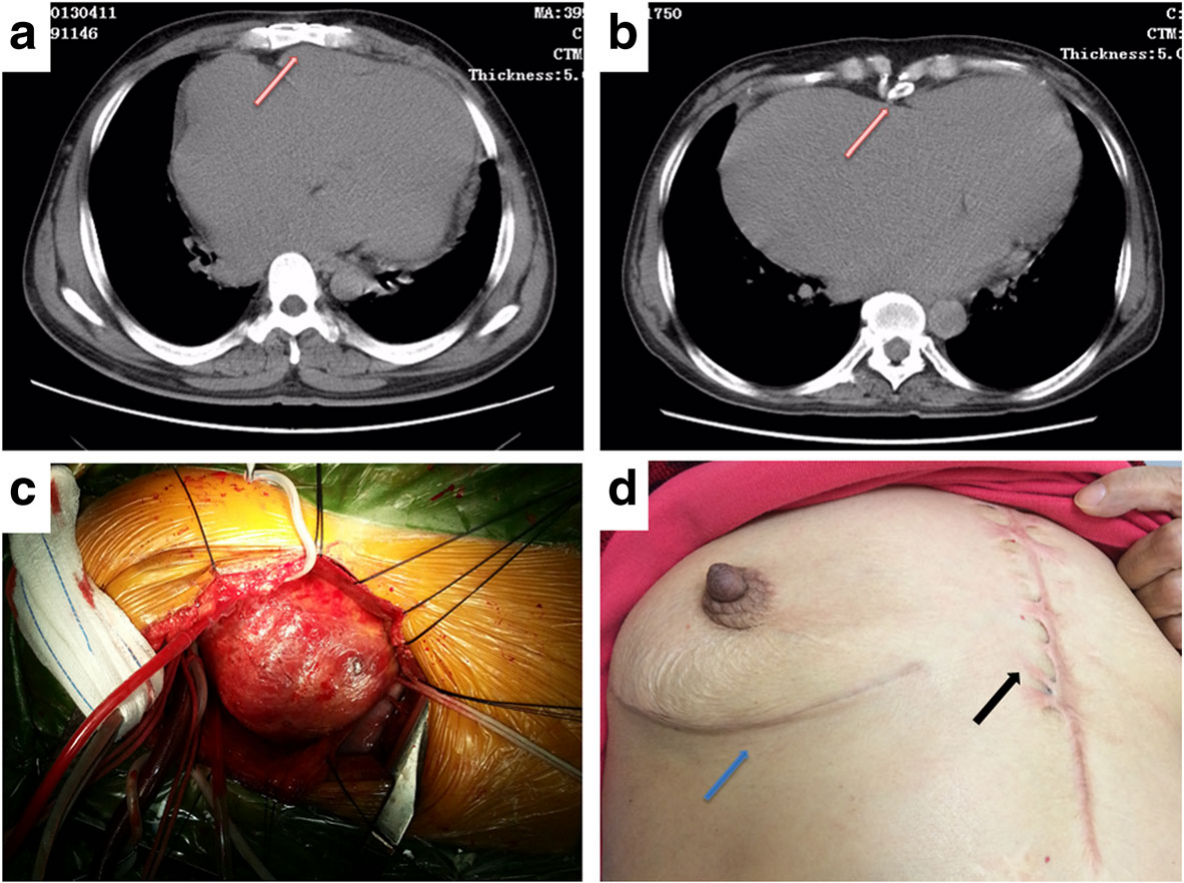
a CT scan shows the firm adhesion between the right ventricle and the sternum in high-risk patients; b CT scan shows the firm adhesion between the right atrium and the sternum in high-risk patients; c The exposure by the right anterolateral thoracotomy after beginning of cardiopulmonary bypass; d The primary incision (black arrow) and the redo incision (blue arrow)
